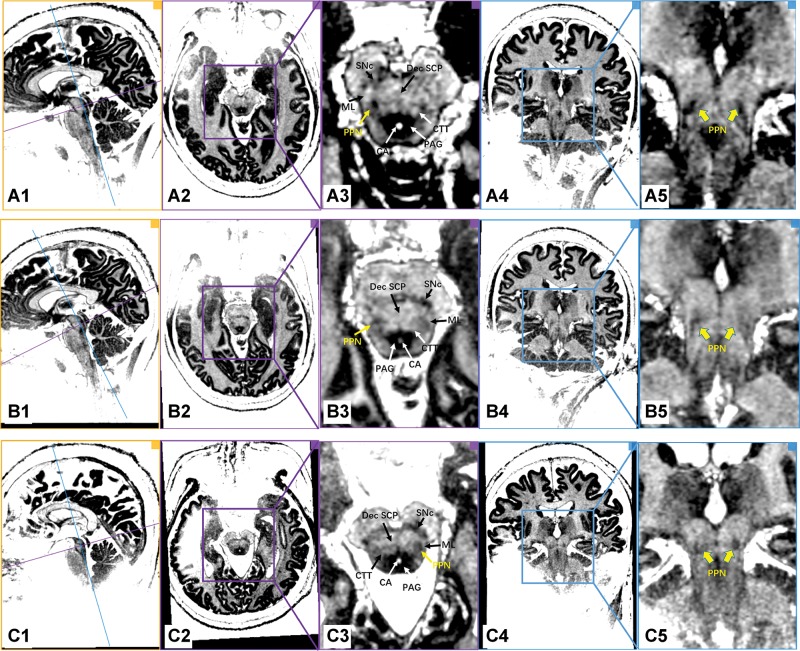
7.0T ultrahigh-field MRI scans using the MP2RAGE sequence in the PD patients (Patients A, B and C). Every row represents the images of the same PD patient. The first column shows the orthographic views for every patient. The two vertically intersecting lines (blue line and purple line) in the first column represent the two planes in other columns (the plane of columns 2/3 with purple border and the plane of columns 4/5 with blue border). The PPN was delineated as a region of intermediate signal intensity when compared to the surrounding structure, such as the hypo-intensity of the PAG and the hyperintensity of the neighboring triad of white matter tracts. Dec SCP: decussating superior cerebellar peduncle, ML: medial lemniscus, CTT: central tegmental tract, SNc: substantia nigra pars compacta, CA: cerebral aqueduct, PAG: periaqueductal gray, PPN: pedunculopontine nucleus.
